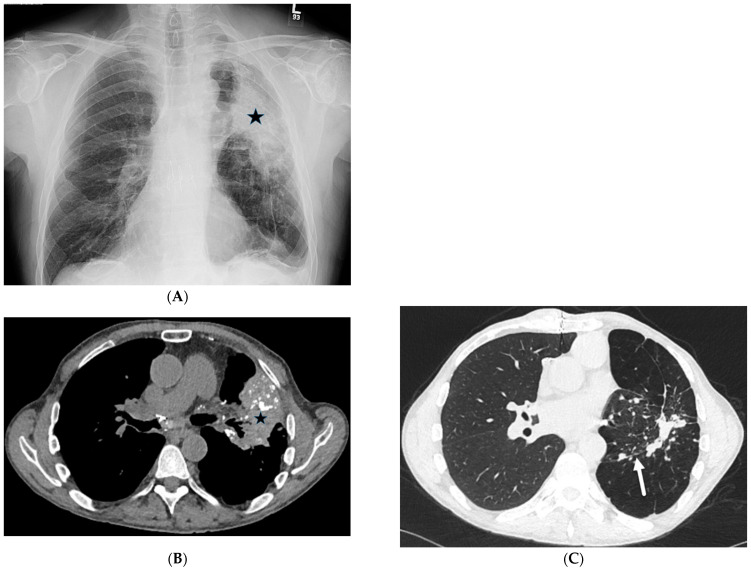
A 65-year-old male with a history of silicosis, coal worker’s pneumoconiosis, and pulmonary hypertension post right lung transplantation. A frontal chest radiograph (A) and axial chest CT images (B,C) demonstrate a partially calcified mass-like consolidative opacity in the left upper lobe (star) representing progressive massive fibrosis. Interlobular septal thickening (solid white arrow) is also noted in the left lower lobe on CT images.
